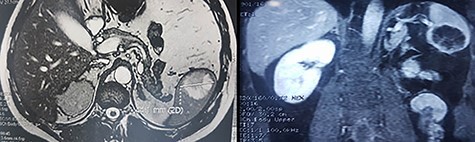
Adominal MRI showing the splenic mass.
